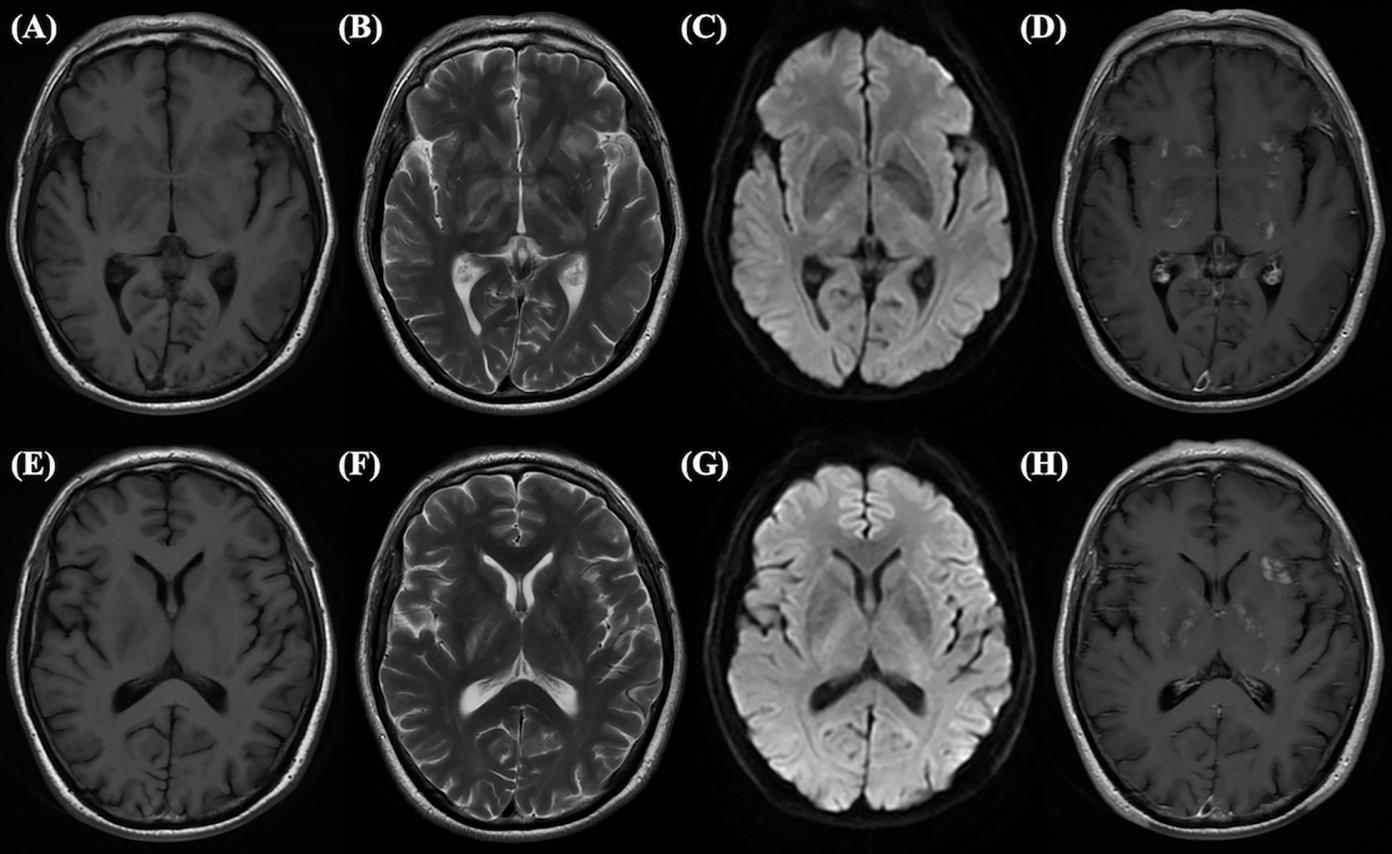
This abnormal MRI is from a 65-year-old male, who tested positive for double antibodies upon first admission. Long signals on T1-weighted (A) and T2-weighted (B) images, slightly high signals on DWI (C), and enhanced shadows on Gd enhancement (D) can be seen in the hippocampus. Long signals on T1-weighted (E) and T2-weighted (F) images, slightly high signals on DWI (G), and enhanced shadows on Gd enhancement (H) can be seen in the basal ganglion.
